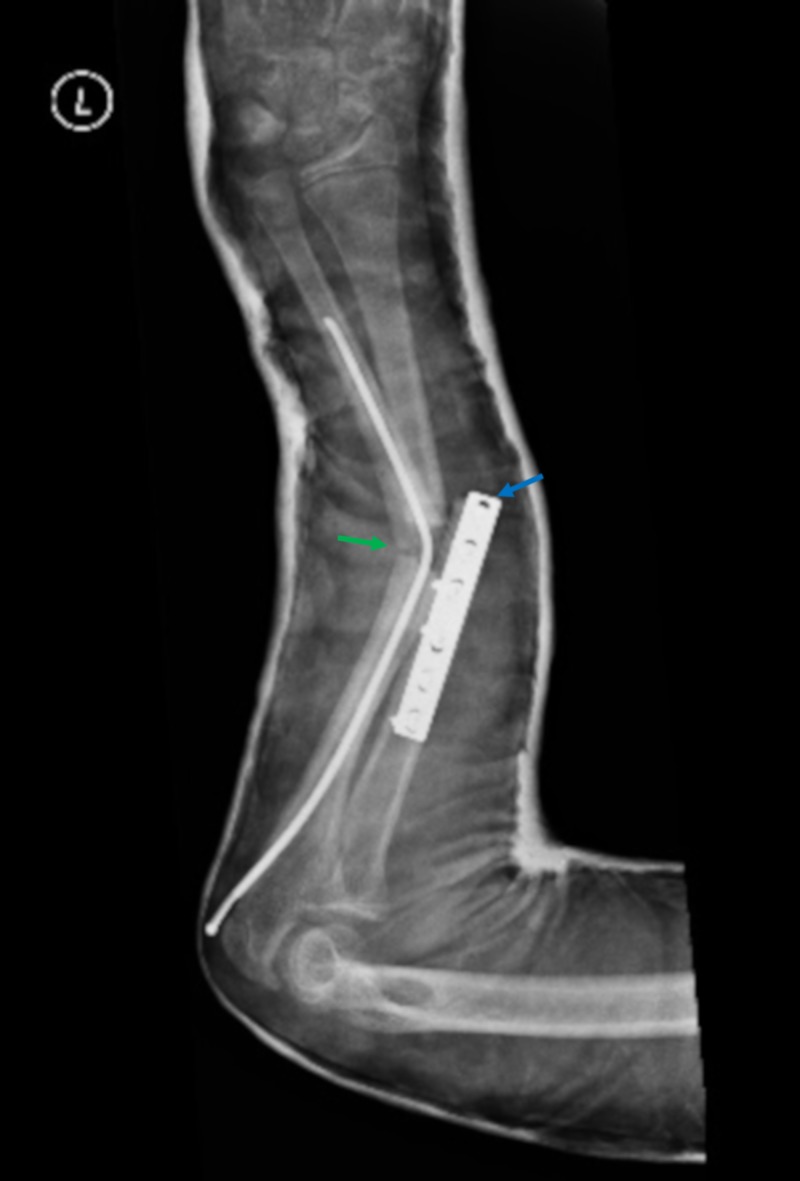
Presenting radiograph Single view radiograph demonstrating plastic deformation of the elastic stable intramedullary nailing in the context of ulna re-fracture (green arrow) and a new fracture at the distal margin of the radial plate (blue arrow).
